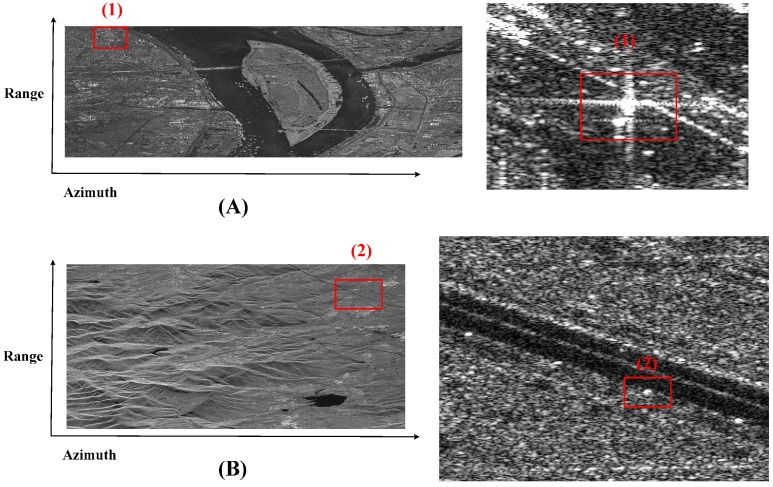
Gaofen-3 1 m resolution sliding spotlight images focusing by proposed Doppler parameter estimation method. (A) Scene 1: the border region between the land and the large-scale sea area. (B) Scene 2: the mountainous area. The strong scattering point is in the square and the enlarged view is beside the picture.
